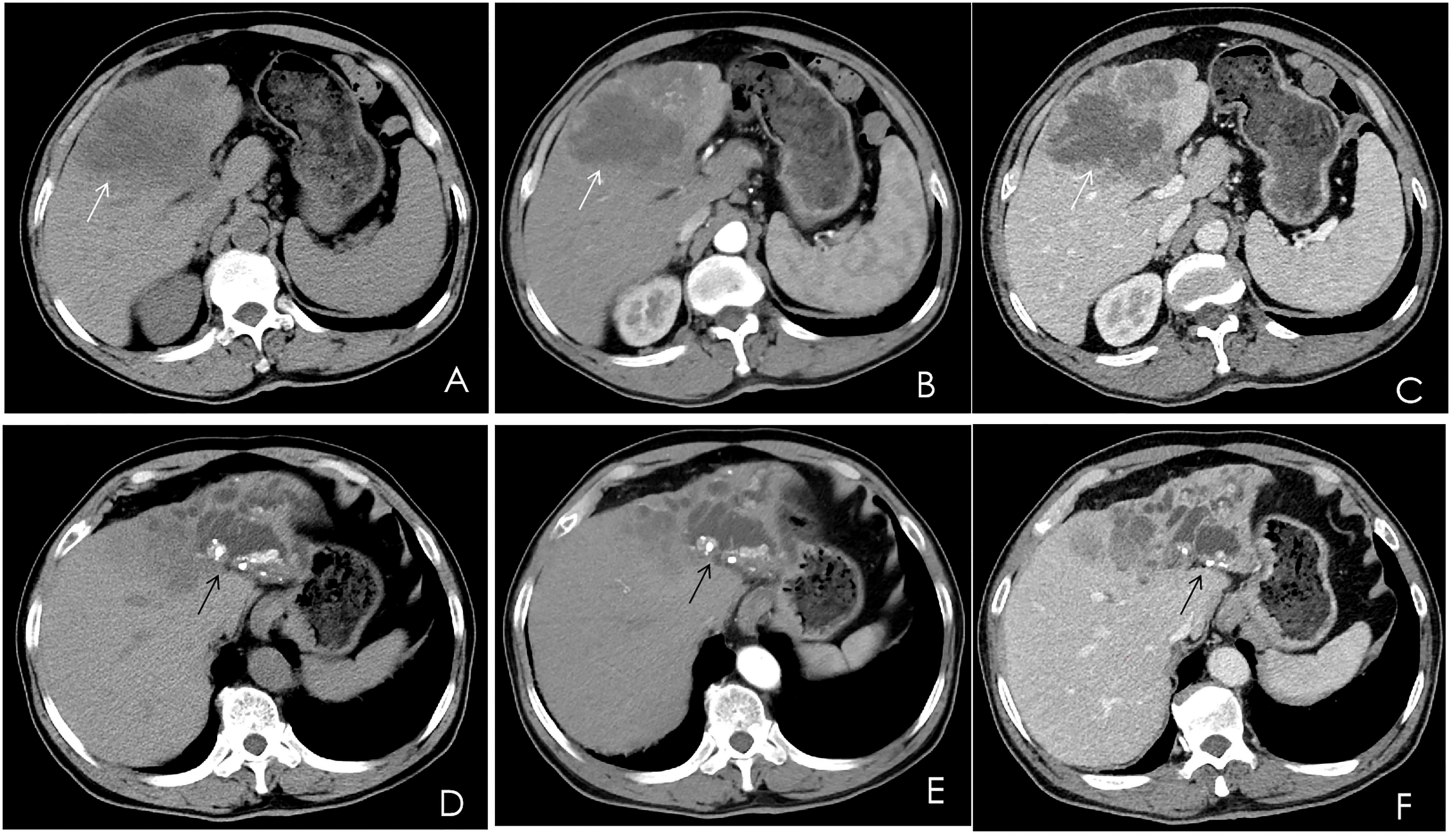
Multiphase contrast-enhanced CT of the upper abdomen, axial view. (A) A noncontrast CT showed multiple low-density masses and nodular mixed-density shadows in the left lobe and part of the anterior right lobe of the liver (white arrow). (B) The arterial phase showed heterogeneous ring enhancement (white arrow). (C) The venous phase showed continuous enhancement (white arrow). (D–F) The left branch of the intrahepatic bile duct and its branches were dilated, and high-density nodular shadows were seen in the liver (black arrow).
